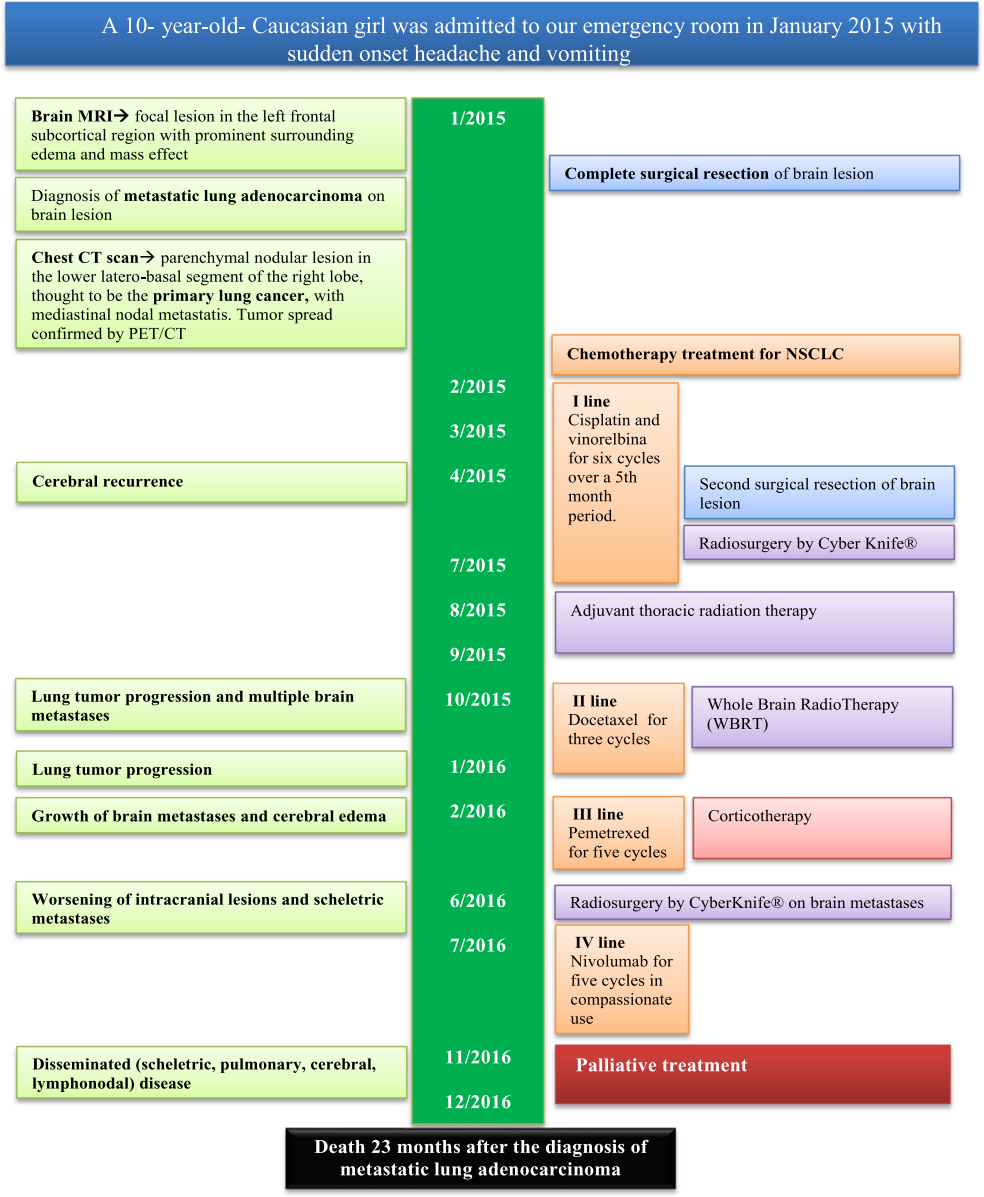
Medical history timeline. *CT* computed tomography, *MRI* magnetic resonance imaging, *NSCLC* non-small cell lung cancer, *PET* positron emission tomography
